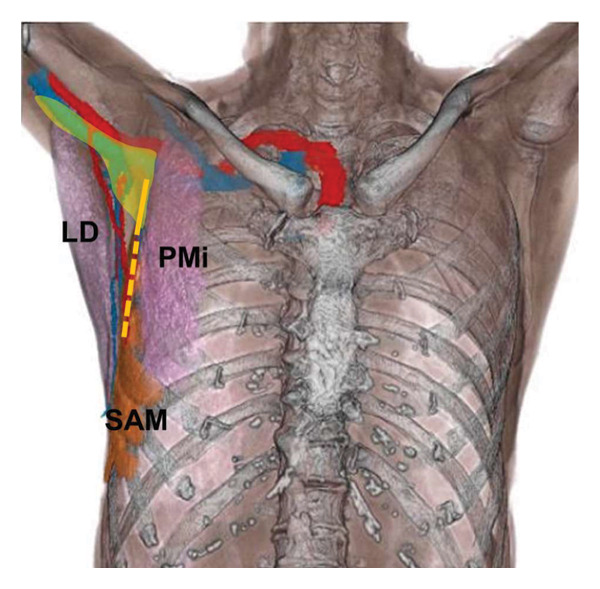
Anatomical relationships of the serratus anterior muscle and surrounding structures reconstructed from the patient’s CT scan. The yellow area shows image of the postoperative spread of contrast medium. The presumed the serratus anterior plane block (SAPB) catheter pathway is shown in orange dashed lines (deep serratus anterior muscle (SAM) layer) and solid lines (migrated to superficial layer). The local anesthetic administered via the SAPB catheter is thought to have spread from the superficial layer of the SAM into the costoclavicular‐SAM space, potentially affecting the medial cord of the brachial plexus. SAM, serratus anterior muscle; PMi, pectoralis minor muscle; LD, latissimus dorsi.
